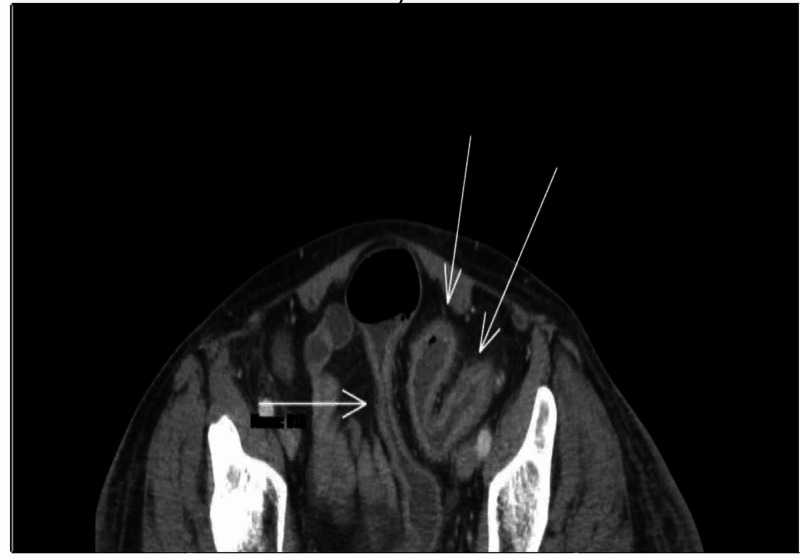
CT scan of abdomen and pelvis showing colitis extending from the hepatic flexure to the rectum. Arrows show mild wall thickening of the sigmoid colon with mucosal hyper-enhancement, mild surrounding fat stranding, and mesenteric hyperemia.
